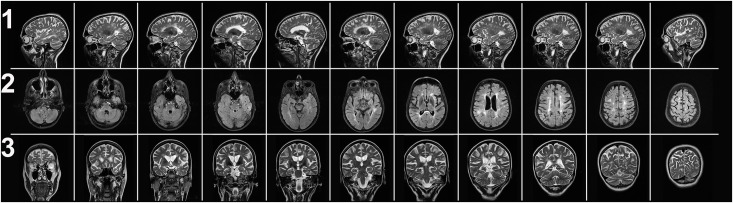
CROP, Clinico-Radiologico-Ophthalmological Paradox in MS. MRI, 3 Tesla, serial sections: 1^st^ row, sagittal, 2^nd^ row, axial and 3^rd^ row, coronar images from a RRMS patient with rather long disease course and highly active disease. Although very high lesion load in the whole brain and visual pathway and obvious brain atrophy the patient had normal RNFL and TMV values. (patient 1, Table 1).
